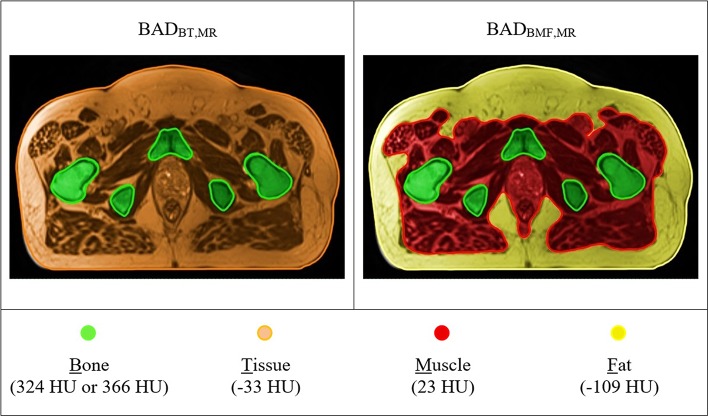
T2-weighted MR based BAD maps with optimal density values. Colors represent the bulk-density assignments on different structures. BAD_BT,MR_ (Bone, B = 366 HU; Tissue, T = −33 HU) and BAD_BMF,MR_ (Bone, B = 324 HU; Muscle, M = 23 HU; Fat, F = −109 HU).
